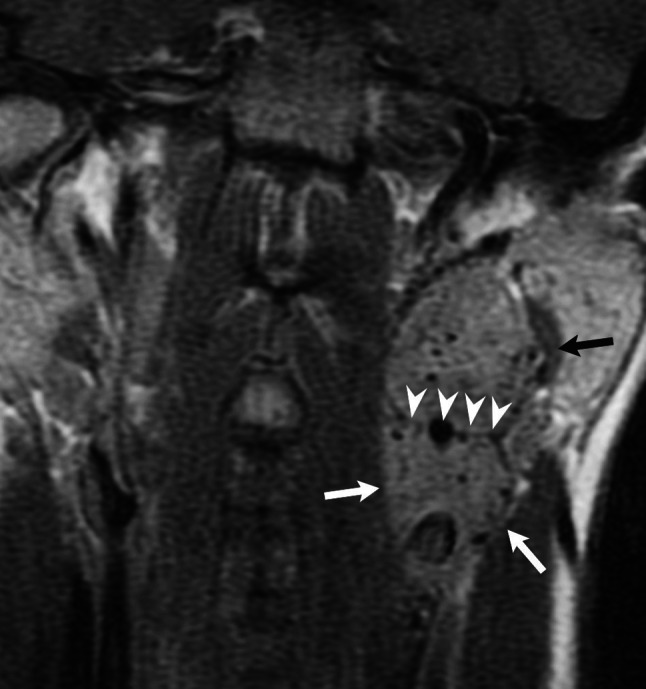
Coronal T1-weighted MR image after application of contrast medium shows thin border (*arrowheads*) between two ipsilateral tumors: vagal PG (*black arrow*) and carotid PG (*white arrows*)
